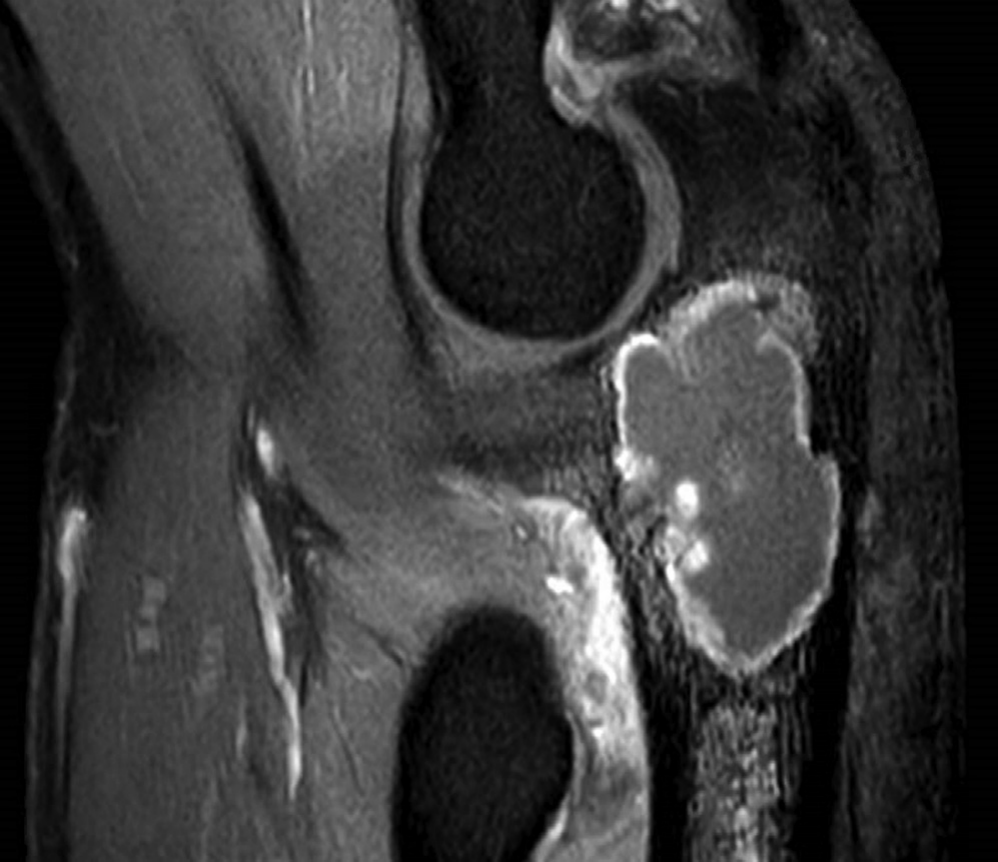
Magnetic resonance imaging scan with gadolinium contrast.
